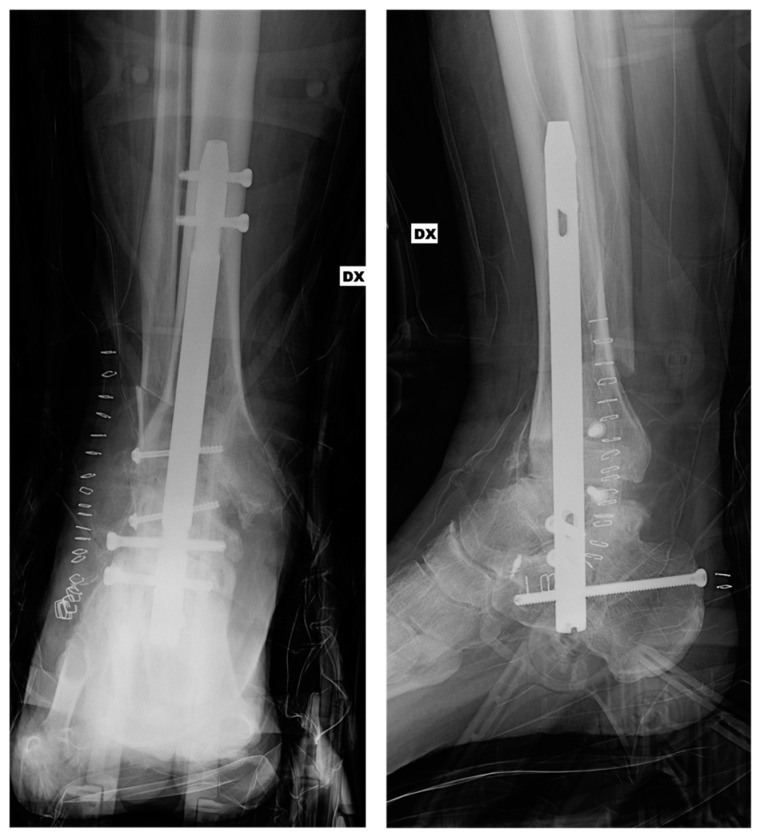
Immediate postoperative anteroposterior (AP) and lateral radiographs.
